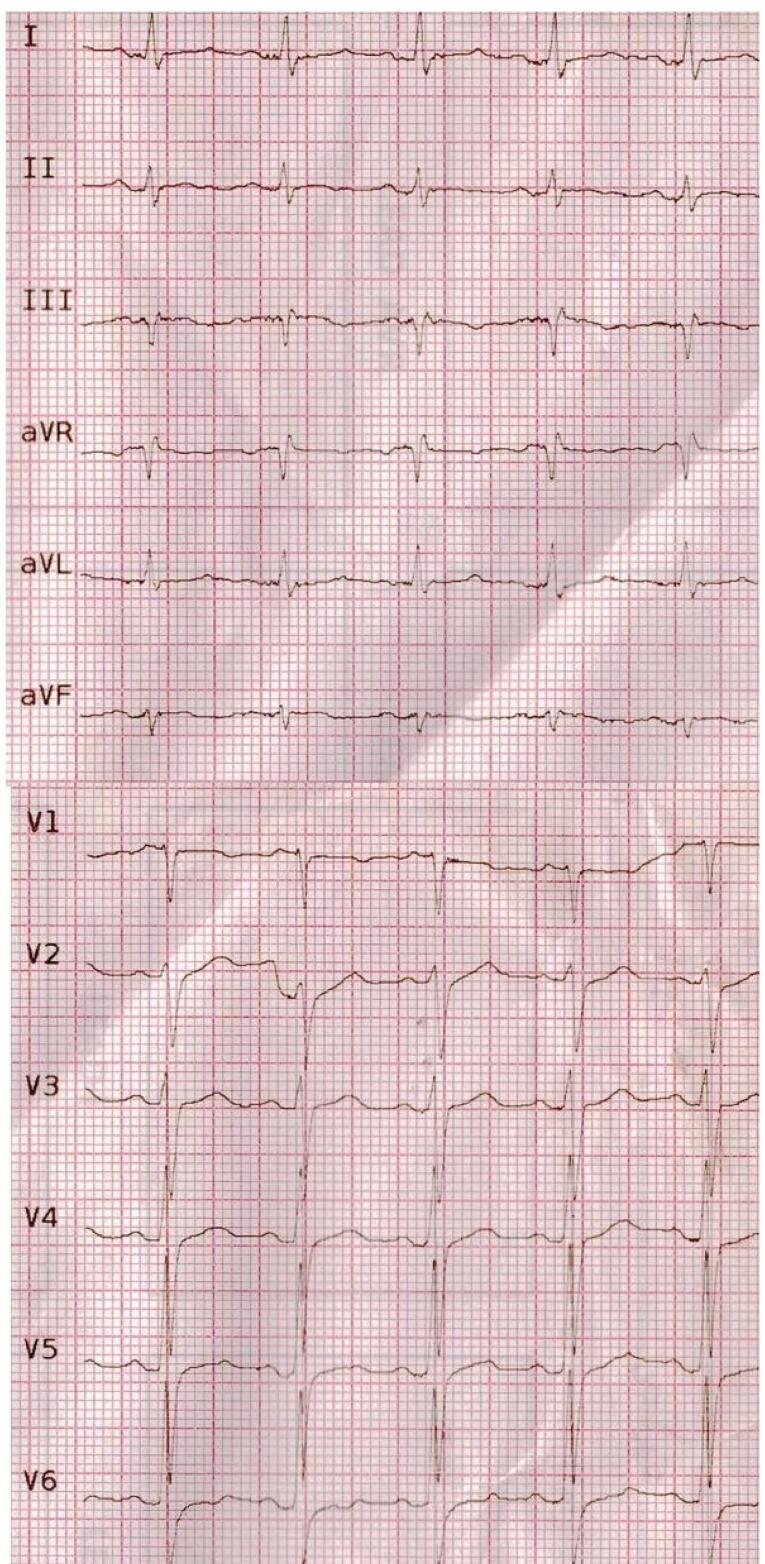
As he was admitted in covid-19 era, spiral lung high resolution computed tomography (HRCT) was done which revealed aneurysmal dilatation of the ascending thoracic aorta of 71 mm diameter and focal hyperdense increased aortic wall thickness.
